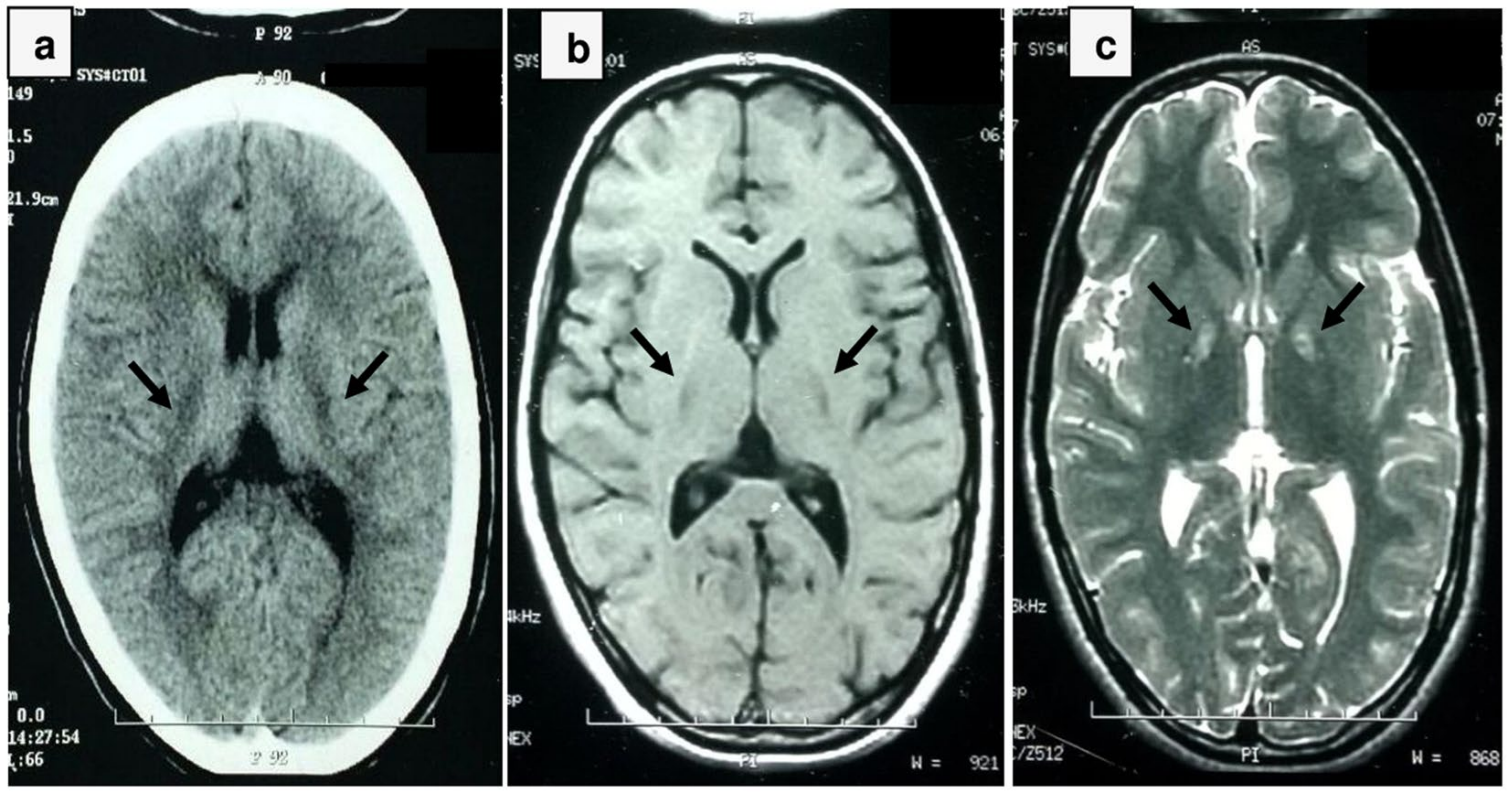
Brain MR-sequences of the proband of the study. Black arrows on the brain MR-sequences of the proband reveal (a) regions of hypointensities in bilateral globus pallidus in brain CT-sequence, (b) regions of hypointensities in bilateral globus pallidus on brain 0.2 T T1W MR-sequence, and (c) regions of hyperintensities in bilateral globus pallidus on brain 0.2 T T2W MR-sequence.
